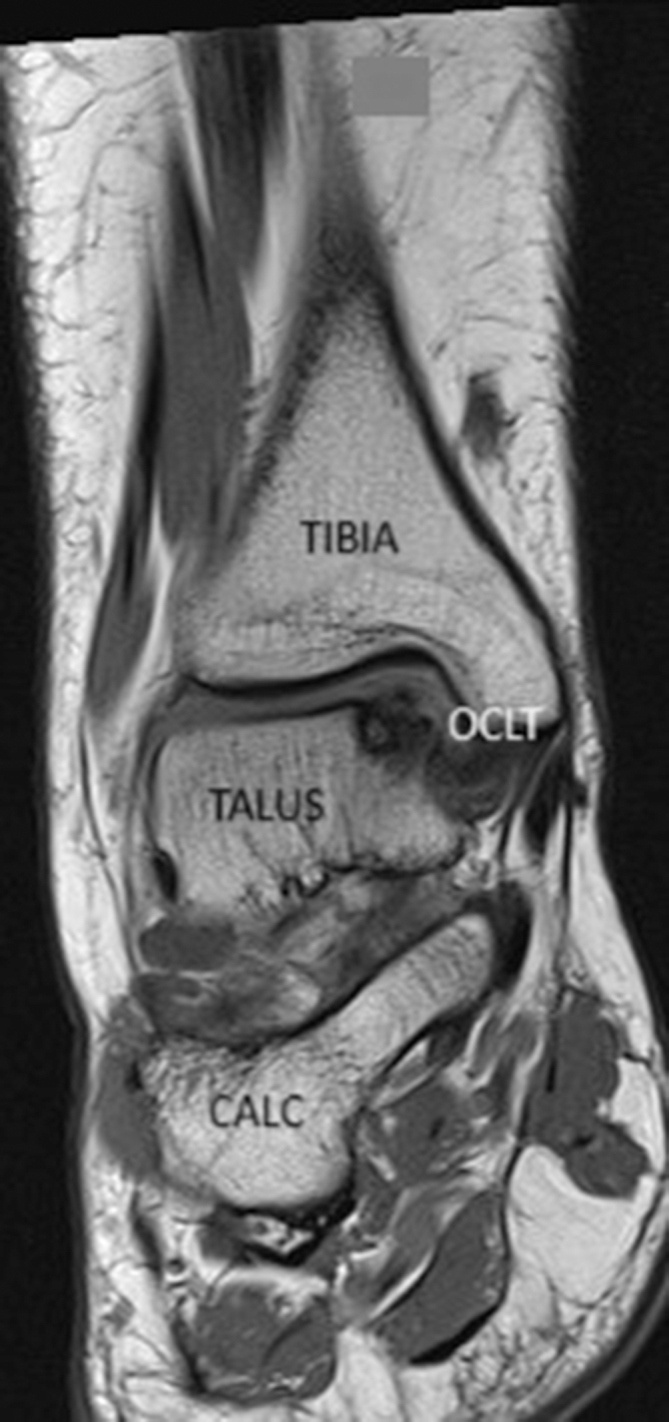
Preoperative anteroposterior magnetic resonance imaging scan showing a medial osteochondral lesion of the talus (OCLT). (CALC, Calcaneus)
